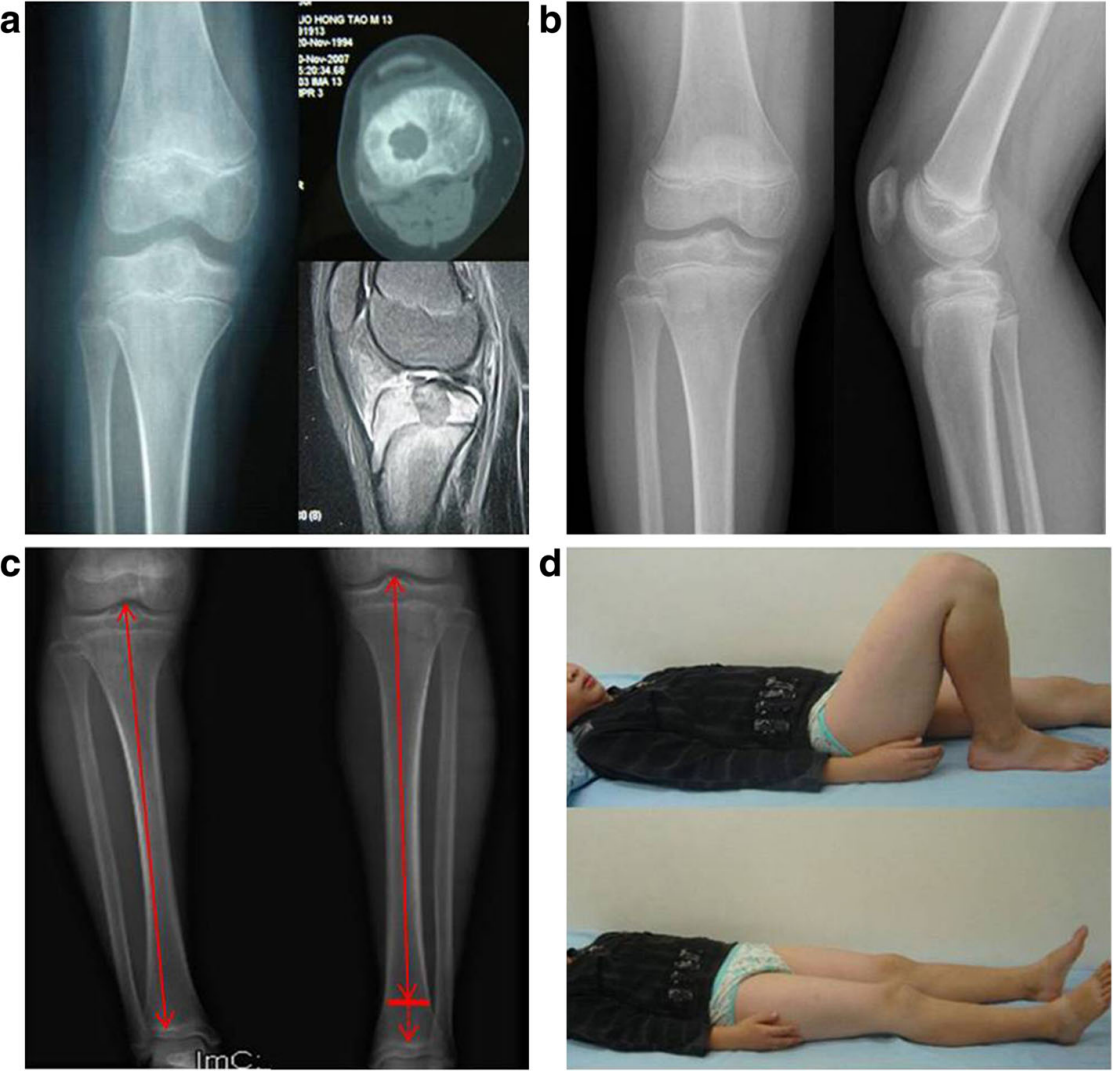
a Preoperative radiographs of a 11-year-old girl. The radiolucent lesion (arrows) and bone cortical erosion in CT and MRI were visible in the proximal tibia. b and c were the X-rays of 3 and 5 years in the course of follow-up. We can see that the density of the tumor cavity become more and more high in the X-ray postoperative. Eight years later, the troubled limb was shorten by 14 mm compared with the healthy limb and mild varus deformity but had a satisfied function of the knee (d)
